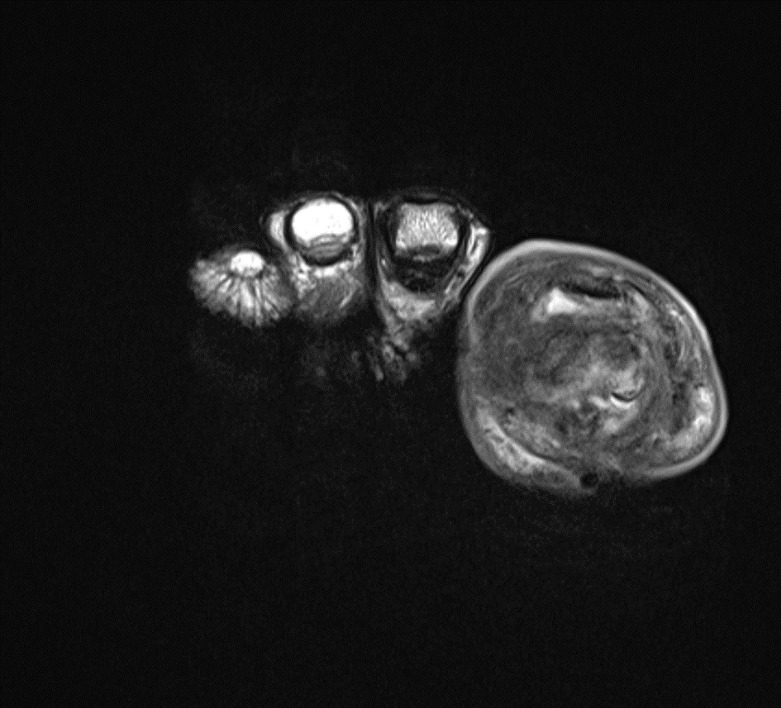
T2-weighted images of the same view as in Fig. 2.
